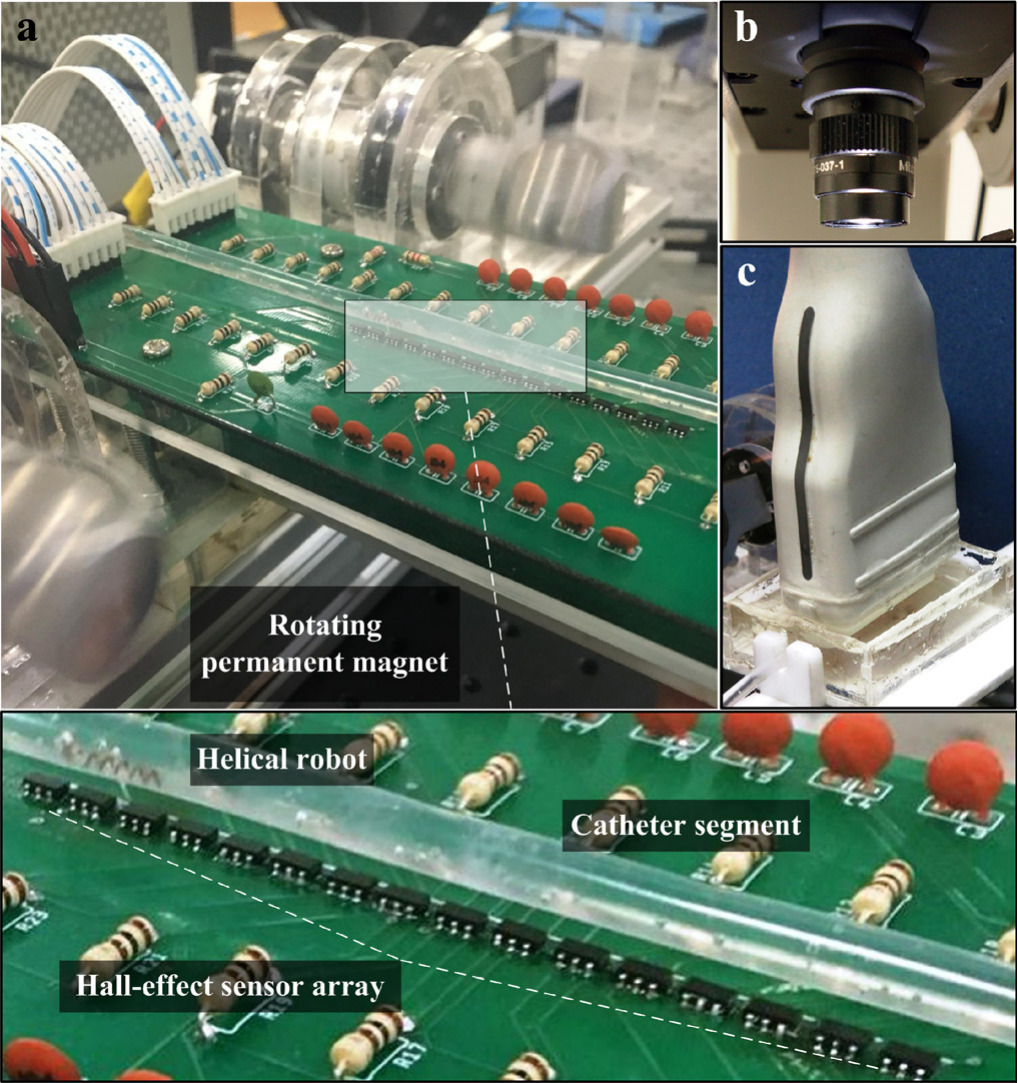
A permanent magnet-based robotic system enables a helical robot to swim using rotating magnetic fields. (a) A catheter segment is aligned with an array of Hall-effect sensors (3D magnetic sensor TLV493D-A1B6, Infineon Technologies AG, Munich, Germany). Position of the helical robot inside the catheter segment is estimated using measurements of these sensors and the precalculated magnetic field map of the rotating permanent magnets. (b) Position of the helical robot is measured with a high-speed camera (avA100-120kc, Basler Area Scan Camera, Basler AG, Ahrensburg, Germany) to validate the magnetic tracking. (c) An ultrasound transducer (LA523 linear array ultrasound transducer, Esaote, Italy) localizes the helical robot inside the *ex vivo* model.
